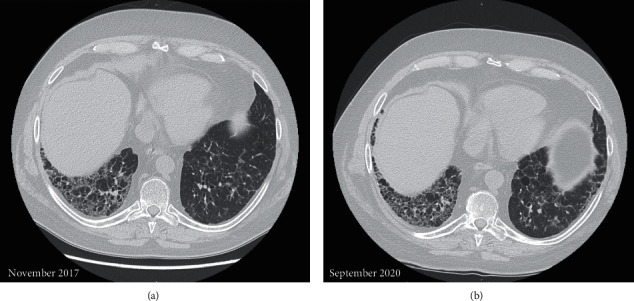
Chest high-resolution computed tomography: November 2017 compared to last follow-up, September 2020. Reticular abnormalities, honeycombing aspects, and traction bronchiectasis compatible with usual interstitial pneumonia. Despite an initial progression, interstitial lung disease showed a substantial stability at the last follow-up.
